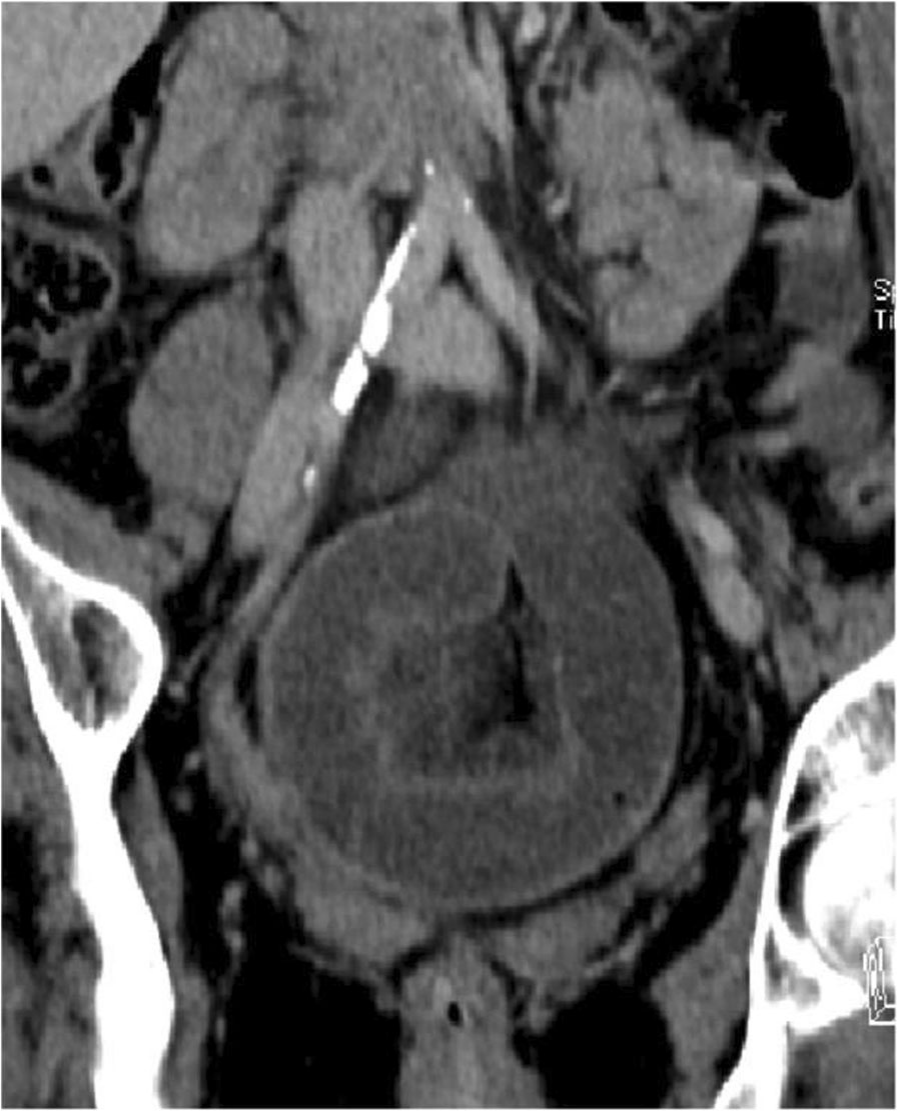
ASBO caused by single band adhesion: CT scan evidence
